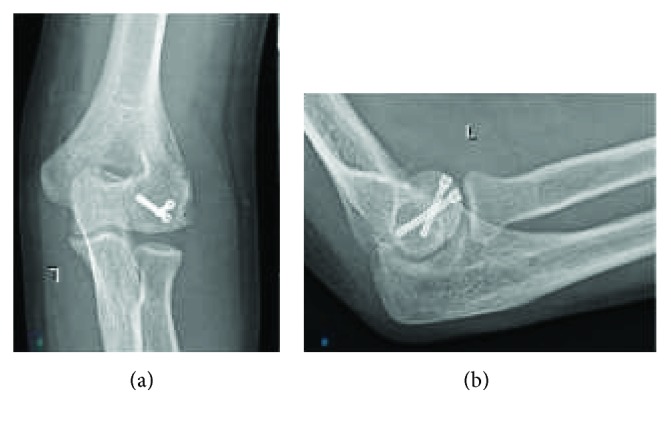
Postoperative control X-rays (third month). (a) Anteroposterior and (b) lateral.
